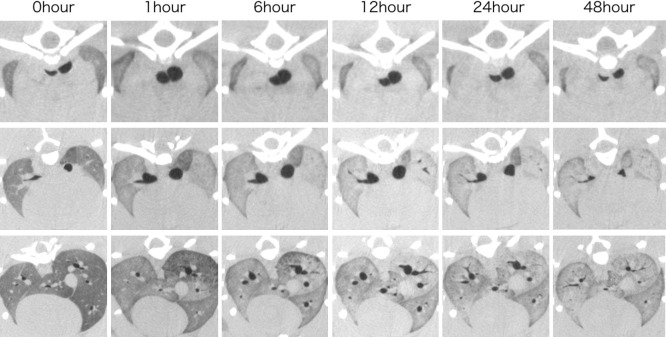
Cross-sectional computed tomography (CT) scan The top image shows the level of tracheal bifurcation, the middle image shows the level of subtracheal bifurcation, and the bottom image shows the base of the lung. Compared with 0 hours, the bronchi expanded 1–12 hours postmortem, then shrank after 24 hours. After 48 hours, the air content decreased and pulmonary edema and congestion were confirmed.
